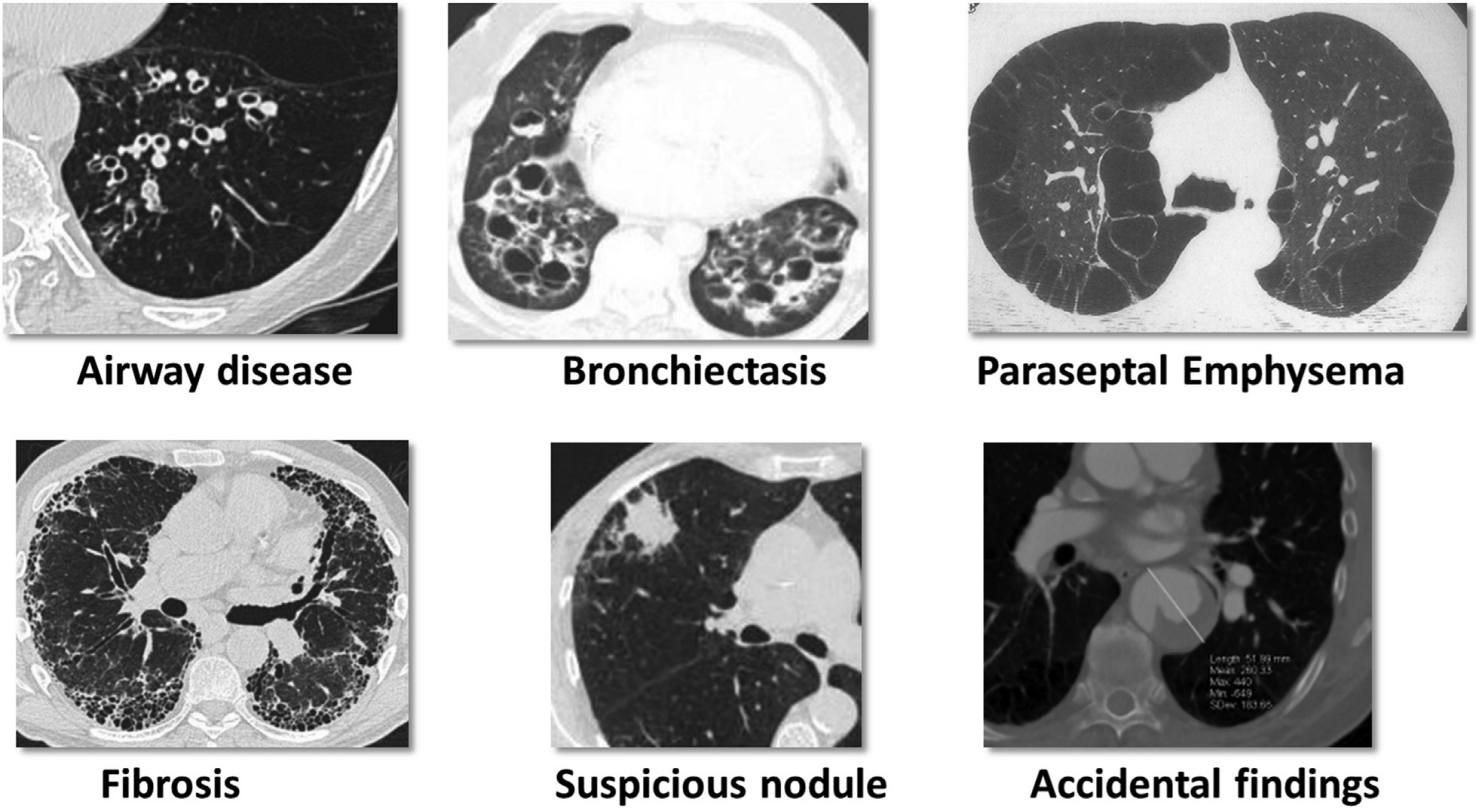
CT findings that exclude patients from endobronchial valve treatment. Each of these findings require a different treatment approach. (Reprinted with permission from Respiration, S. Karger AG, Basel, Switzerland).^4^
